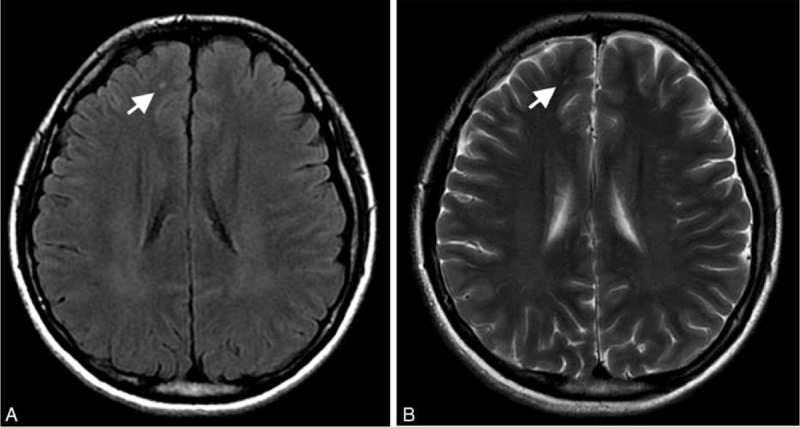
Axial T2 FLAIR (A), T2WI (B) of a 29-year-old female patient with HNPP. Hyperintense focus (white arrow) is observed in subcortical white matter of right frontal lobe on T2 FLAIR and T2WI.
